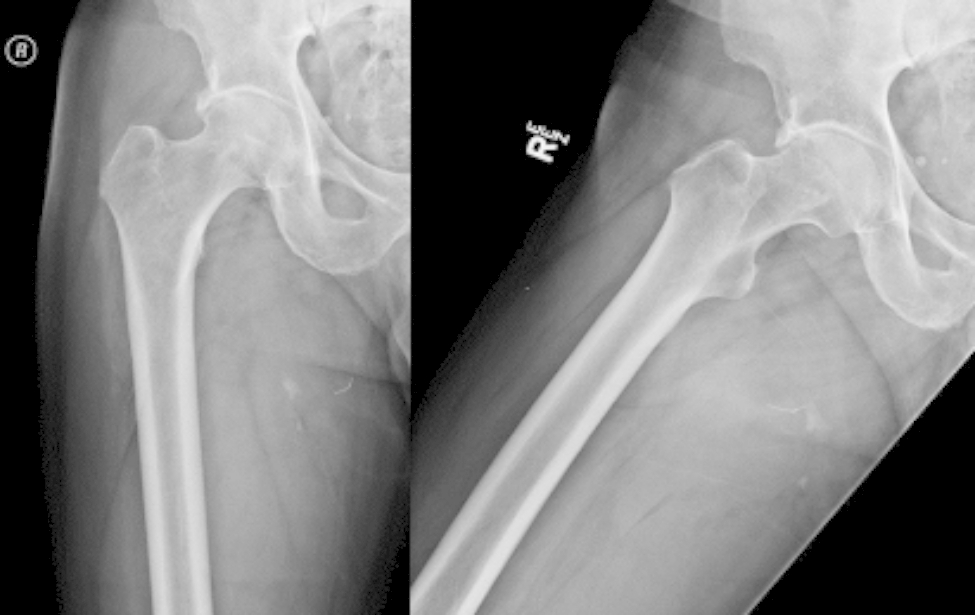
Initial anterior-posterior (left) and lateral (right) radiographs of the right hip
